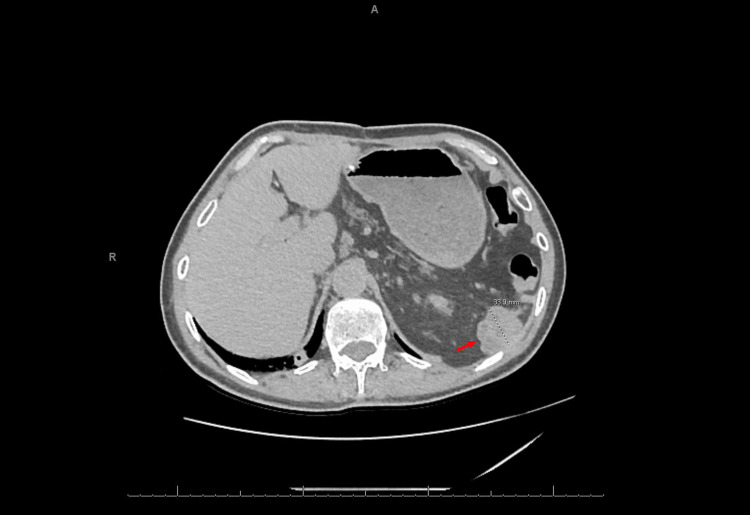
CT scan of the abdomen and pelvis without contrast (axial view) showing a lobulated mass in the splenectomy bed (arrow). Pathological evaluation of the mass demonstrated a malignant solitary fibrous tumor. CT: computed tomography
